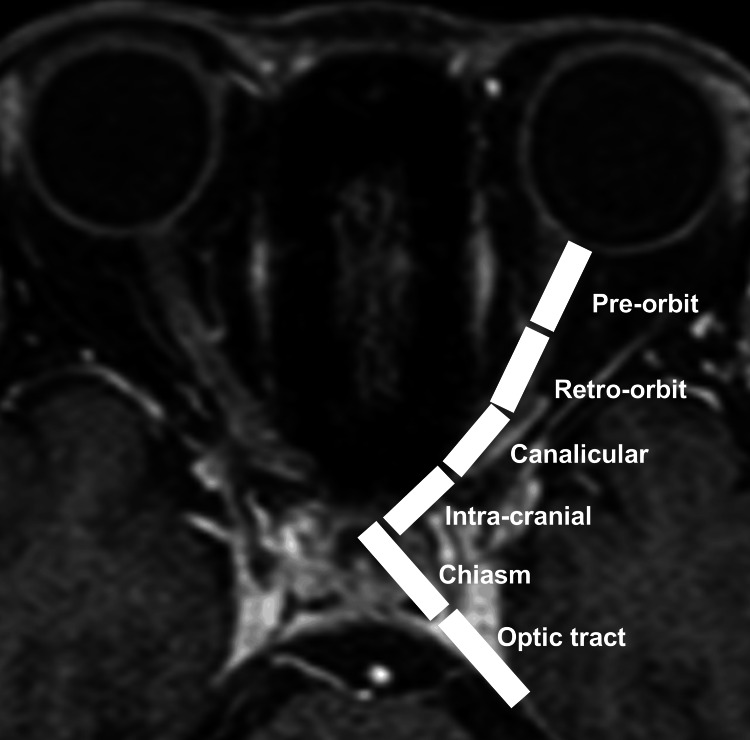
Definition of the MRI lesion segments. The optic nerve was divided from anterior to posterior by pre-orbit, retro-orbit, canalicular, intra-cranial, chiasm, and optic tract.
